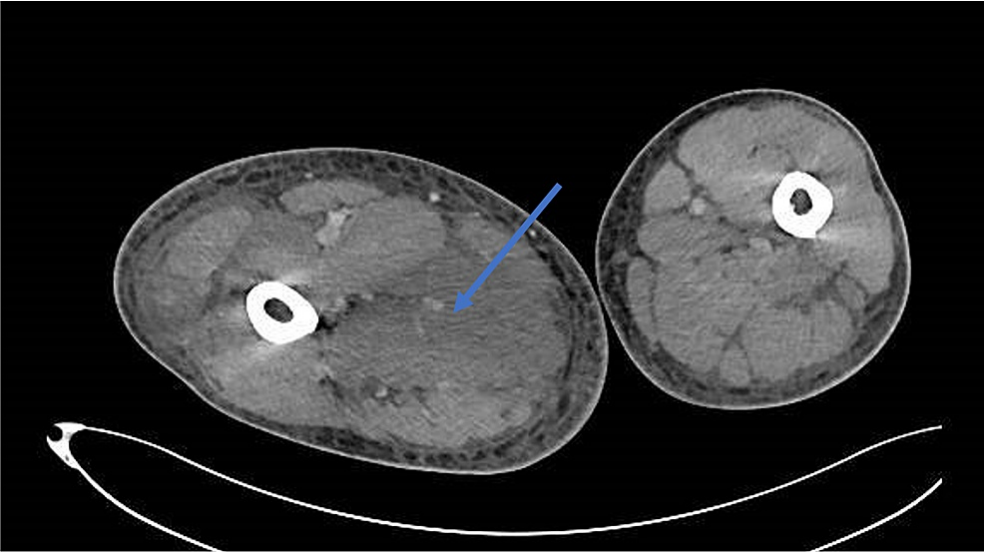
CT scan of the right thigh revealing soft tissue edema involving the muscular planes, particularly in the adductors compartment (arrow) compatible with myositis. CT, computed tomography
